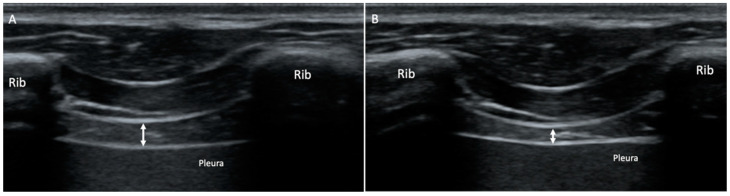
B-mode ultrasound imaging of the diaphragm thickness showing the last intercostal space at the mid-axillary line from the upper edge of the 12th rib to the lower edge of the 11th rib of the thorax. (A) Diaphragm thickness measurement marked by a white arrow at maximum inspiration (T^ins^) during normal breathing. (B) Diaphragm thickness measurement marked by a white arrow at maximum expiration (T^exp^) during normal breathing.
